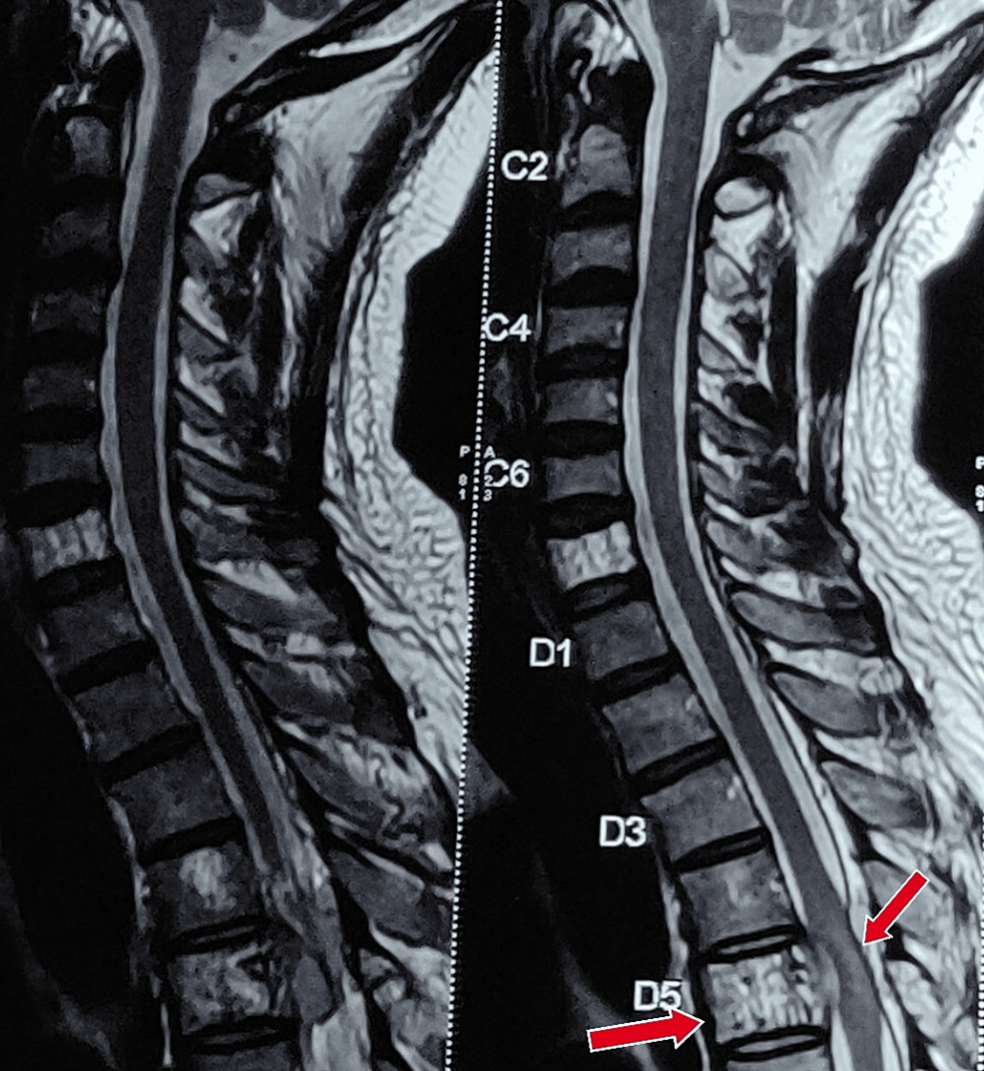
The MRI shows heterogeneously enhancing altered marrow signal noted in the D5 vertebral body There is an enhancing epidural soft tissue component noted at the D5 level causing moderate narrowing of the central canal with compression of the dorsal cord MRI: magnetic resonance imaging
